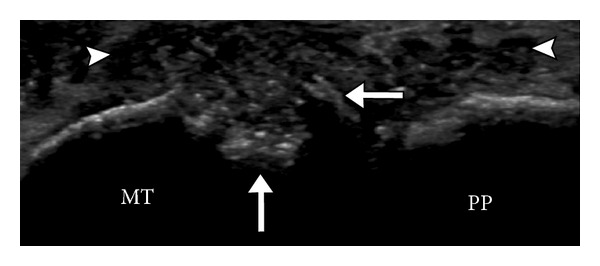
Synovitis and erosion. Ultrasound appearance of erosion in the metatarsal head demonstrates cortical irregularity, focal defect, and overhanging edge (arrows) with adjacent synovitis (arrowheads). MT: metatarsal; PP: proximal phalanx.
